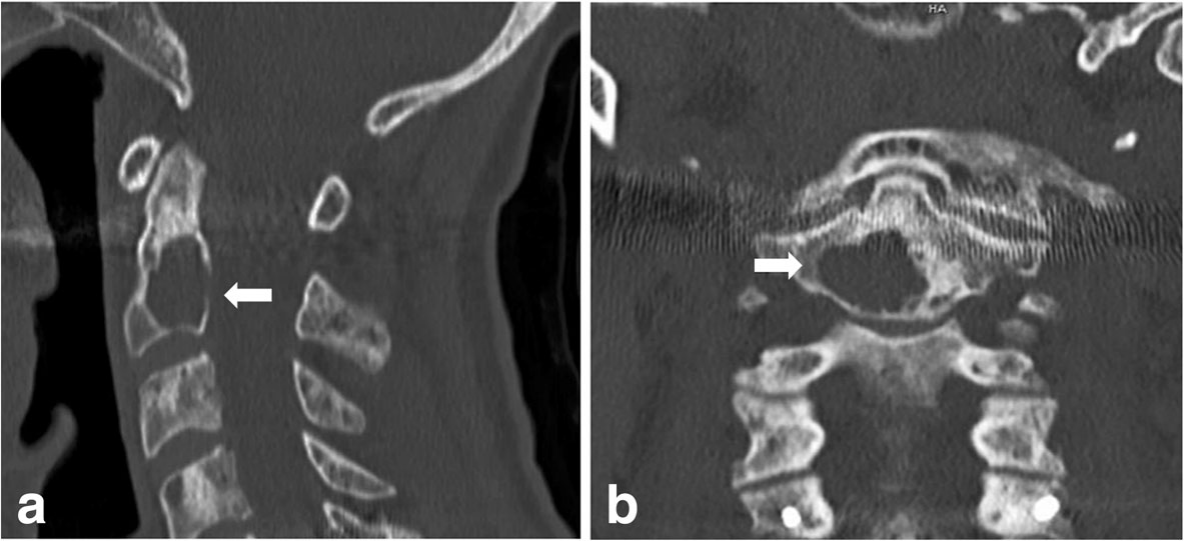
CT-scan in lateral (a) and coronal (b) view with instable osteolytic lesion (arrows) in the base of C2 (16 mm × 11 mm × 15 mm) (Case 1)
